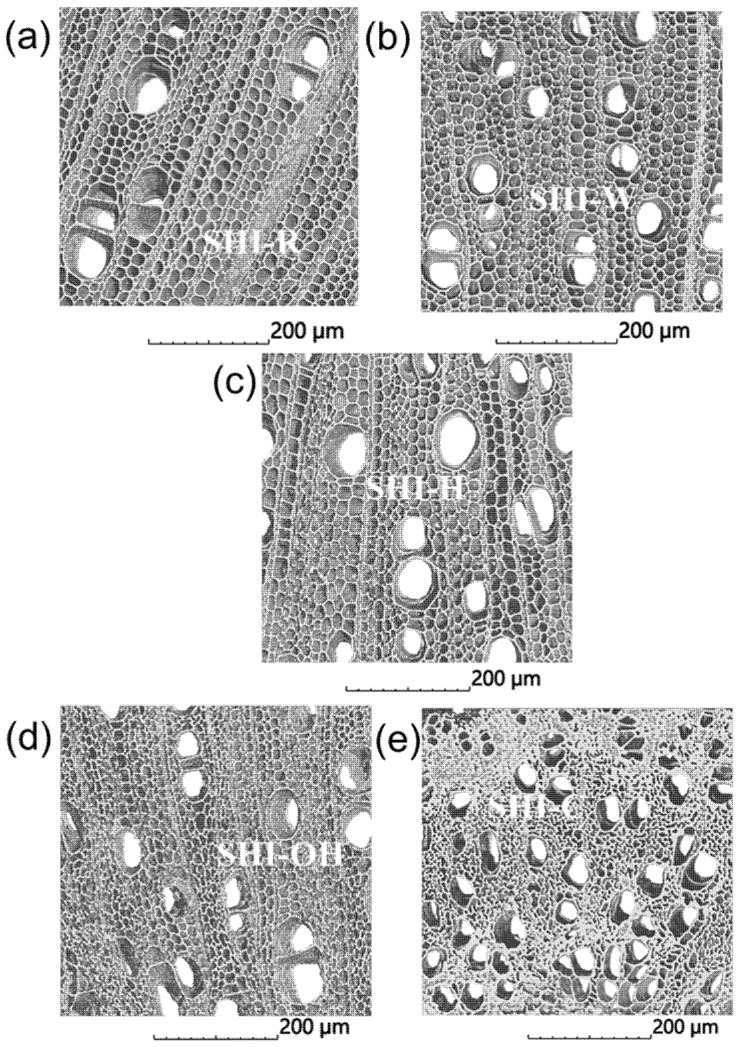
X-ray computed nanotomography images of untreated SHI-R (a) and treated SHI-W, SHI-H, SHI-OH and SHI-C (b–e) hemp shive samples.
